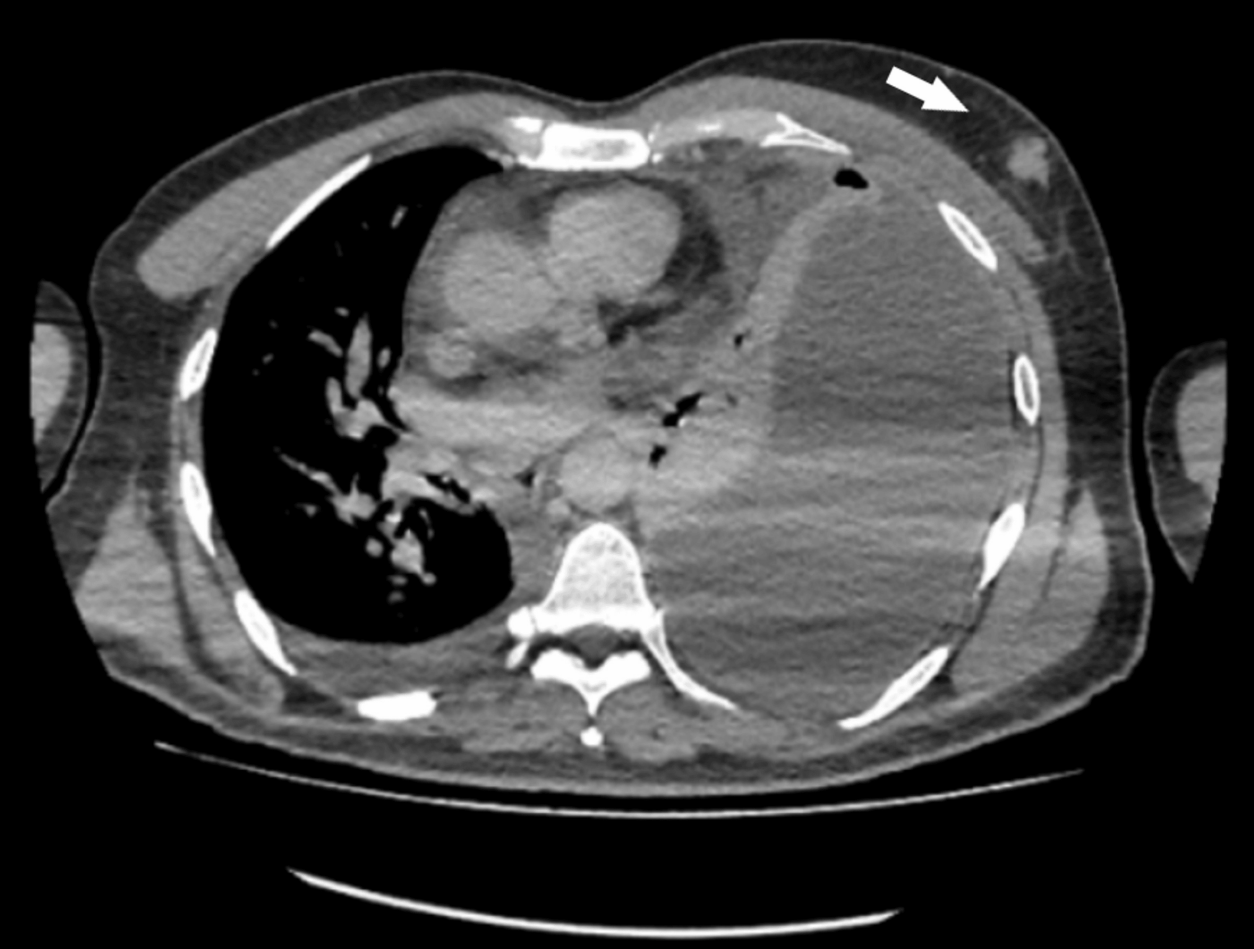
Axial CT scan of the chest. Axial CT image of the chest demonstrates a lobulated nodule with spiculated margins in the upper outer quadrant of the left breast, measuring 1.7 × 1.4 cm, adjacent to a large left-sided pleural effusion.
